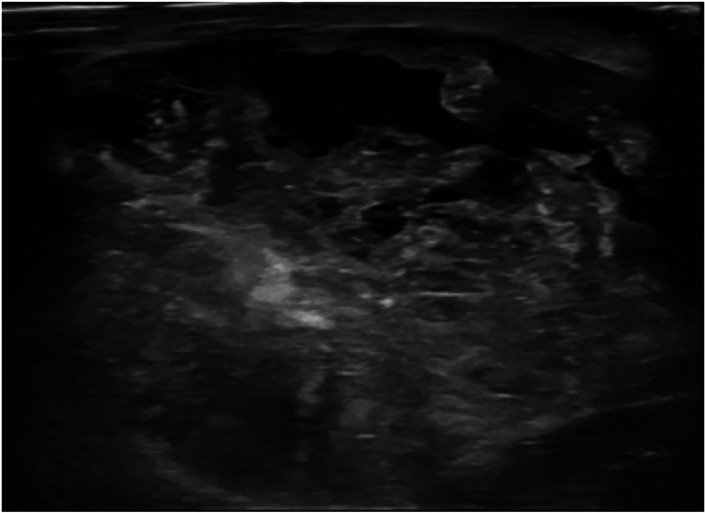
A 42-year-old woman with malignant phyllodes tumor. The ultrasound showed a huge circumscribed heterogeneous echoic mass with internal cystic spaces involving the entire right breast.
